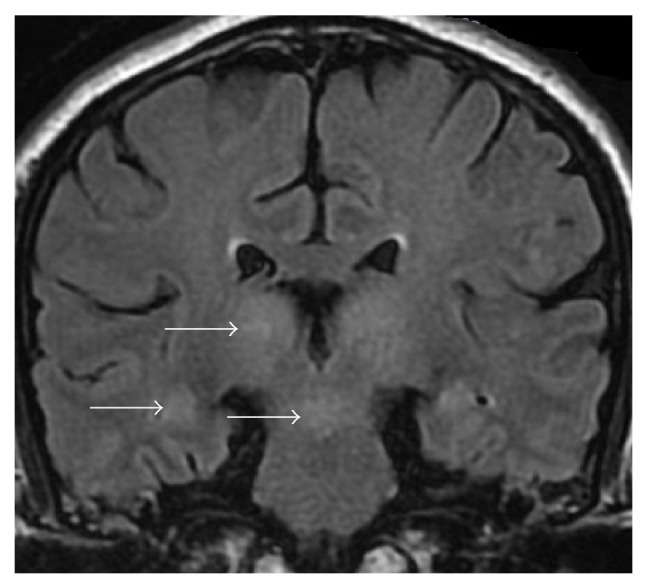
There are widespread abnormal signals at bilateral thalamus and hippocampus on FLAIR image.
